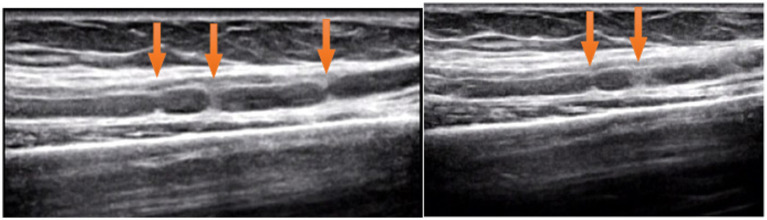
The longitudinal ultrasound image shows multiple HLCs of AIN (arrow).
